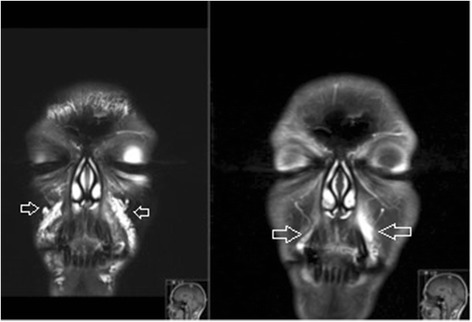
Hyaluronic acid dermal filler in the lower infraorbital triangles (*open arrows*) on coronal MRI (T2FS)
